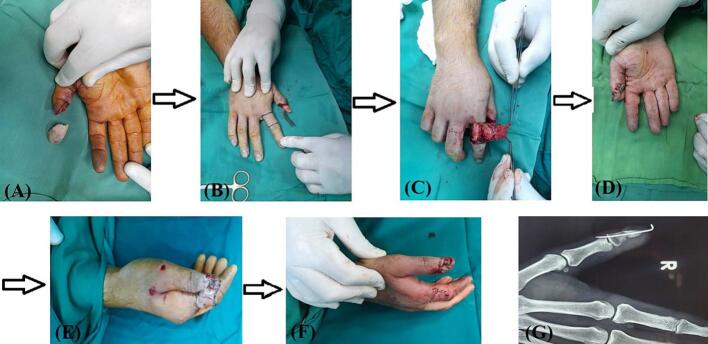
(A) Describing the procedure for replanting a distal thumb following a sharp, oblique amputation using an adiposofaciocutaneous flap technique. (B) Marking the flap area on the proximal part of the index finger for two transverse incisions. (C) and (D) Detaching the adiposofaciocutaneous flap with an intact base from the side of the index finger and suturing it rolled around the thumb. (E) Covering the defect on the dorsal side of the index finger with a full-thickness graft from the volar side of the same wrist, connecting the adiposofascial part to the cutaneous part from the dorso-radial side. (F) Dressing the wound with Vaseline and moist gauze, removing sutures after two weeks, and detaching the flap's base from the index finger after three weeks. (G) A plain radiography (P-A) after 4 weeks.
